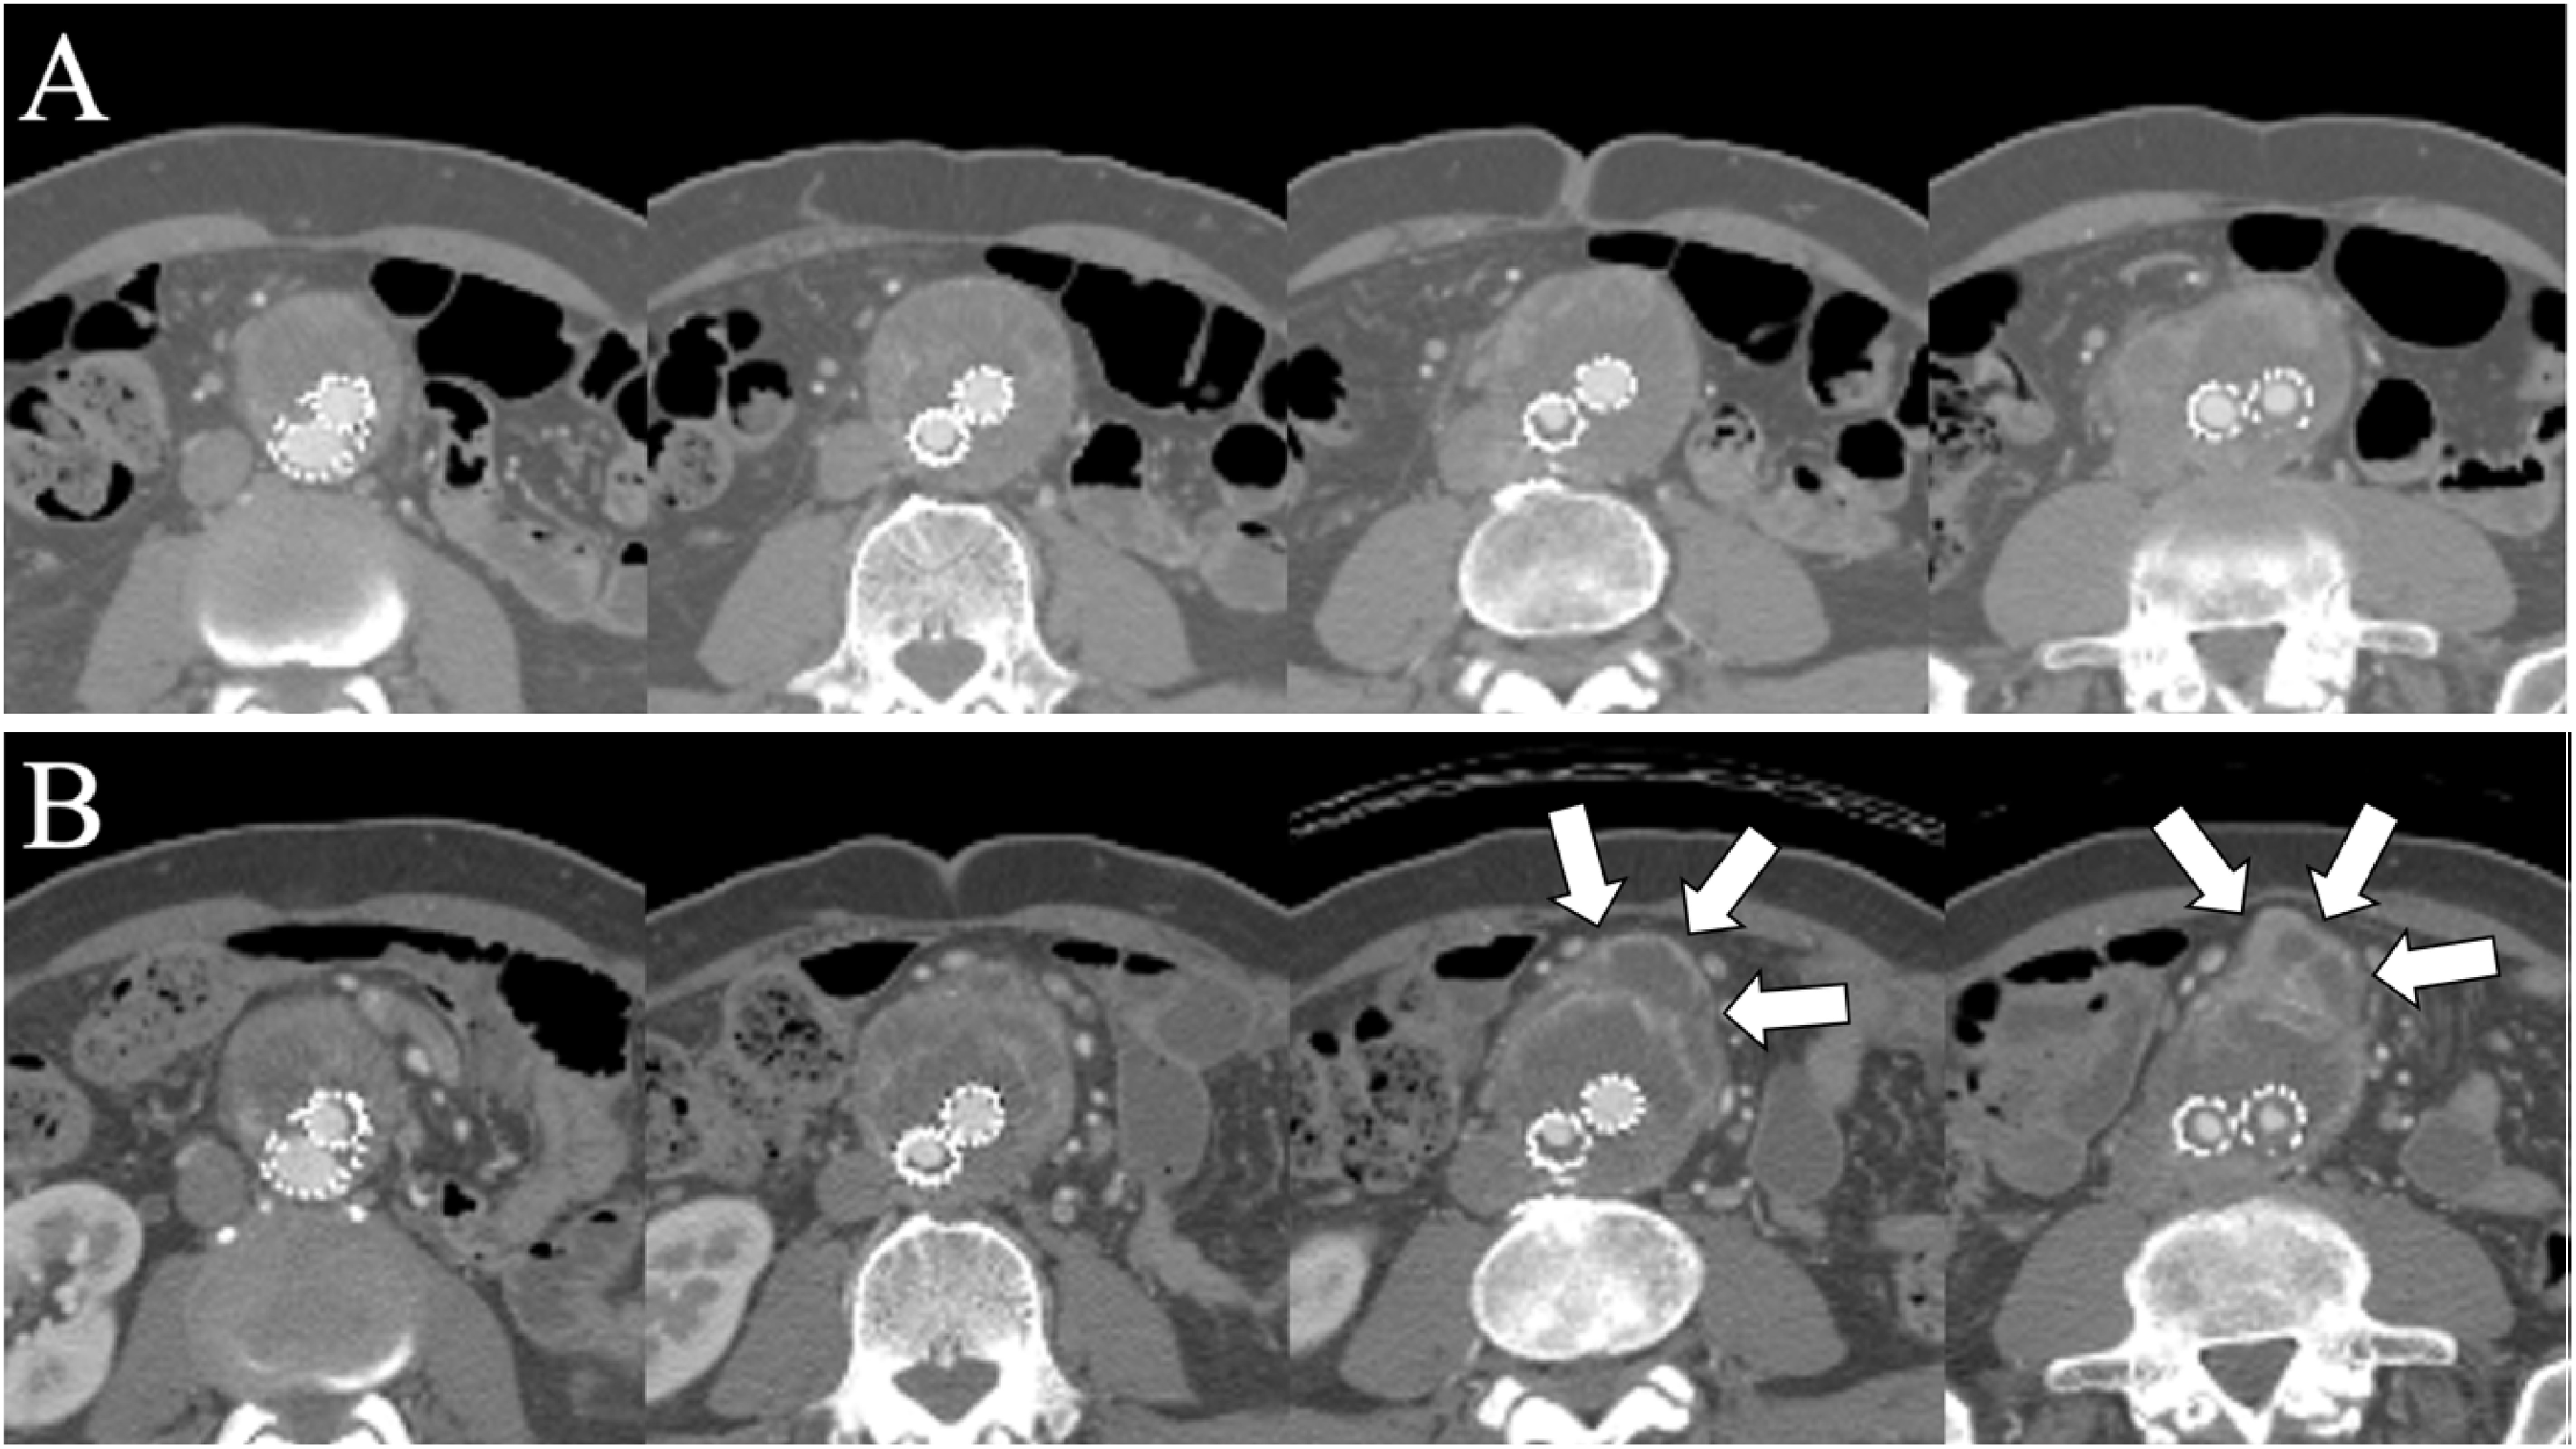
Fig. 1 Serial computed tomography (CT) image of aortic aneurysm follow-up. (A) CT surveillance scan four months before the patient became symptomatic. (B) CT scan at the time of presentation with an increase in aneurysm size from 4.8 to 5.5 cm, periaortic thickening, and periaortic inflammation (arrows). There was no endoleak during observation.
